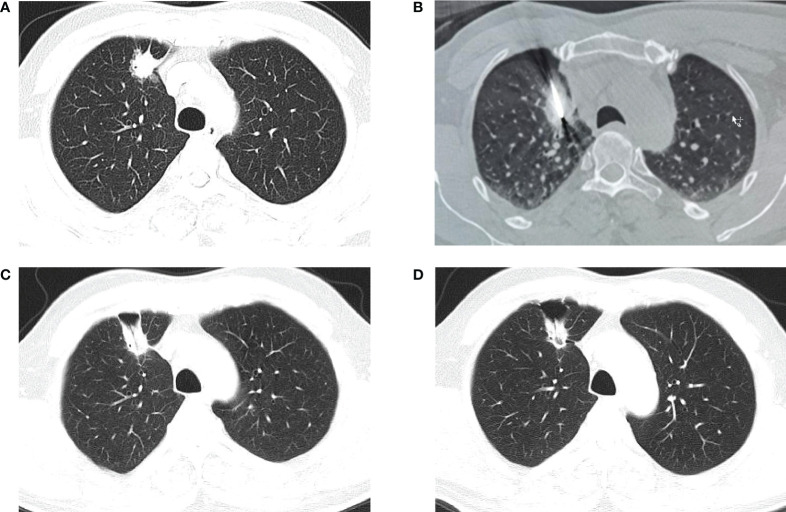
CT-guided percutaneous MWA. (A) CT scan performed before MWA, showing a lesion in the upper right lobe. (B) CT−guided percutaneous MWA of the lesion. (C) CT scan performed 1 month after the procedure. (D) CT scan performed 4 months after the procedure.
